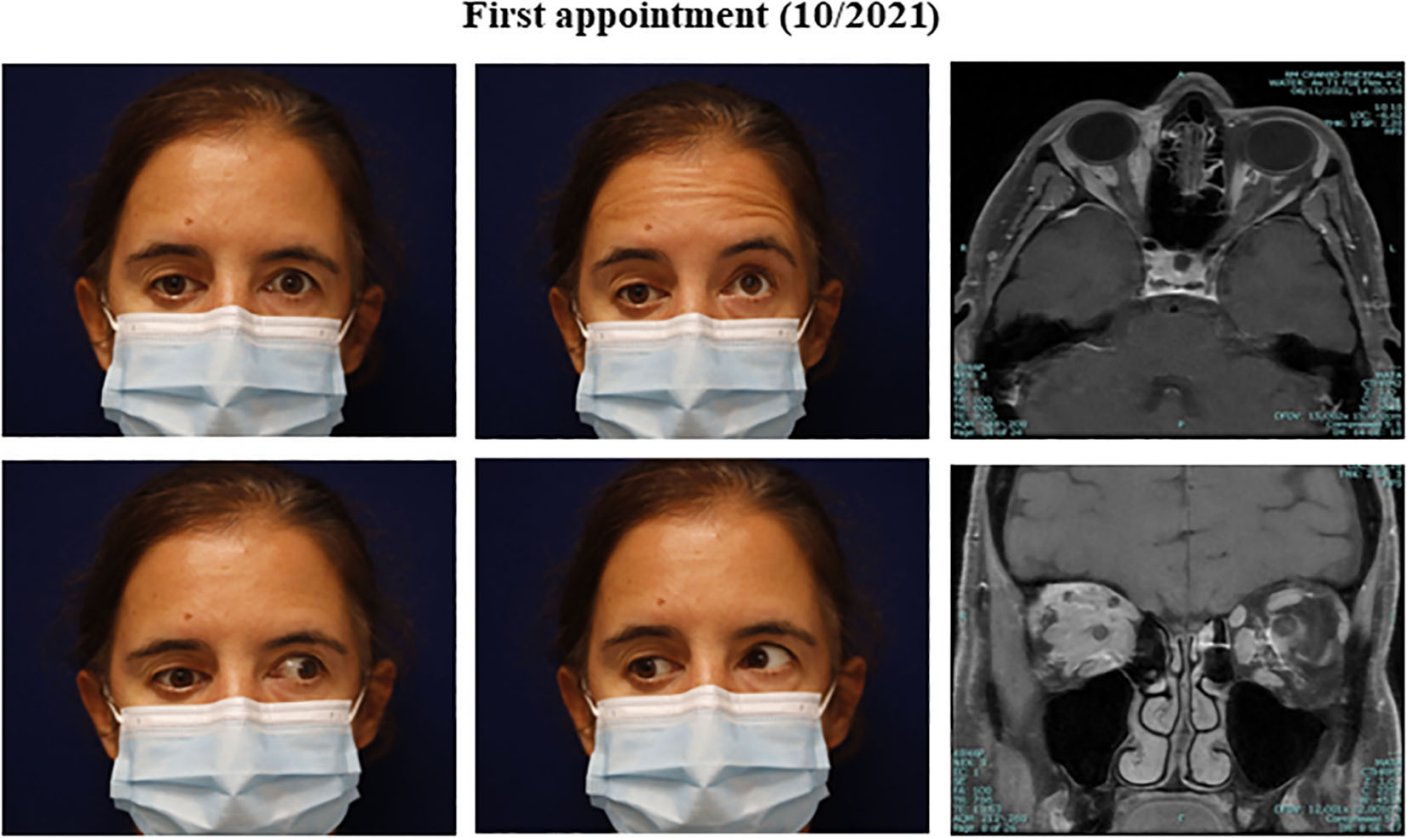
Clinical presentation and orbital findings at the initial appointment. (Clinical pictures) Right inferior dystopia with restriction in extraocular elevation and adduction of the right eye. (Orbit Imaging) Orbit axial and coronal T1 MRI showing post-gadolinium enhancing lesions (intra and extra-conal), with mass effect and inflammatory changes of orbital fat.
